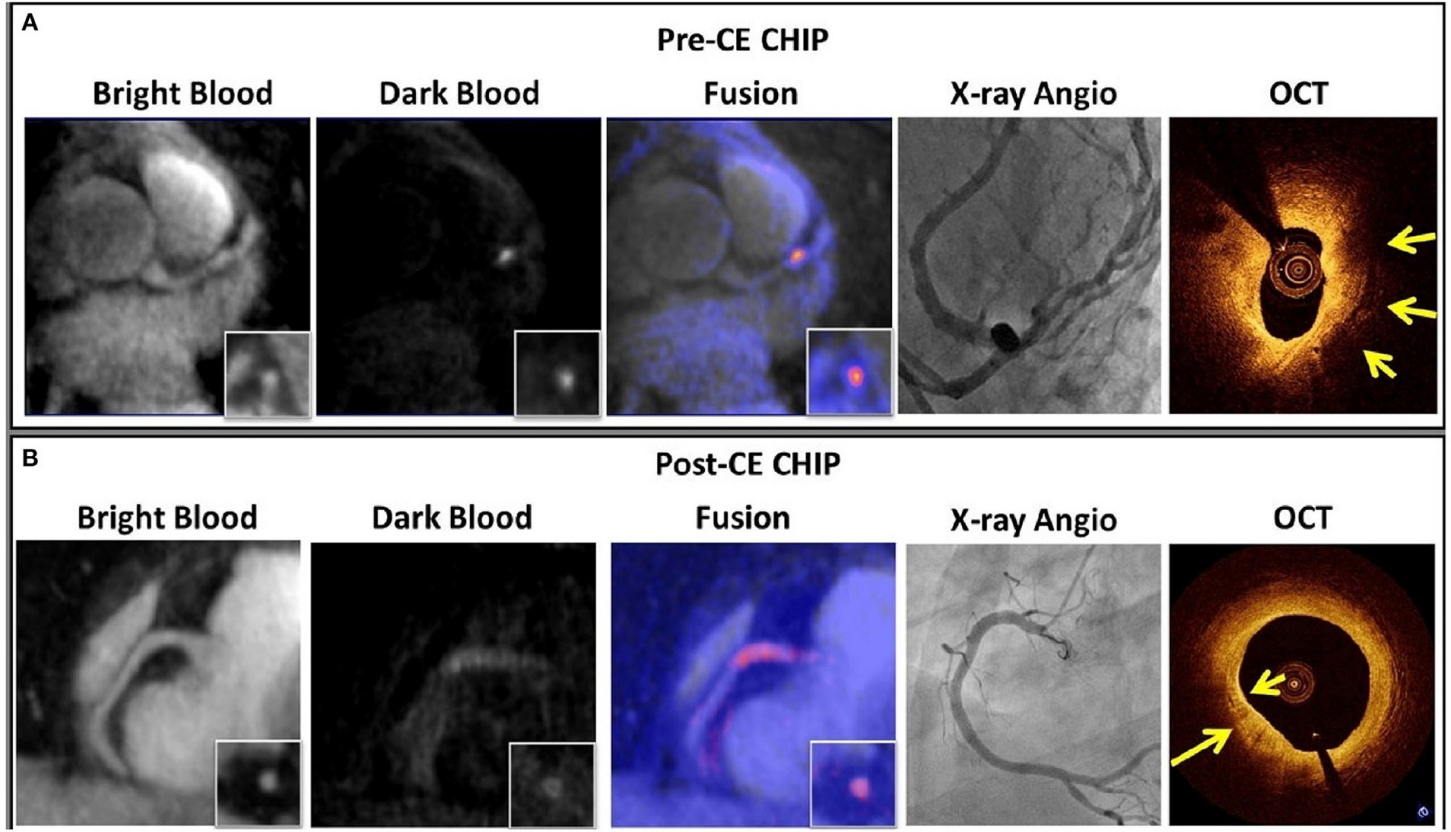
(A) An example of pre-CE CHIP was found in the middle LAD visualised on the dark blood images and fused with the bright-blood scan. XA showed significant stenosis (70%) at that location. OCT showed large signal-poor area suggestive of possible lipid core and/or intra-plaque haemorrhage (yellow arrow). (B) An example of post-CE CHIP with diffuse wall enhancement at proximal RCA as localized on the bright-blood images. XA showed only mild stenosis (30%) at that location. OCT showed strong multi-focal back reflections and signal heterogeneity within the overlaying tissue suggestive of high macrophage density (yellow arrows). CE, contrast enhancement; CHIP, coronary hyper-intensive plaques; XA, X-ray angiography; OCT, optical coherence tomography. Adapted with permission from Xie et al. (96).
